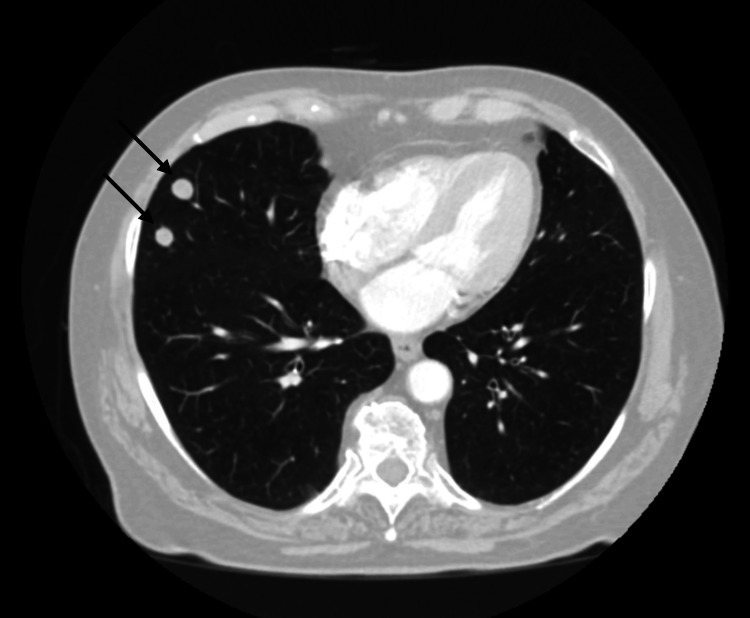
Initial CT of the chest, abdomen, and pelvis, which showed two right middle lobe nodules, concerning for the metastatic process
